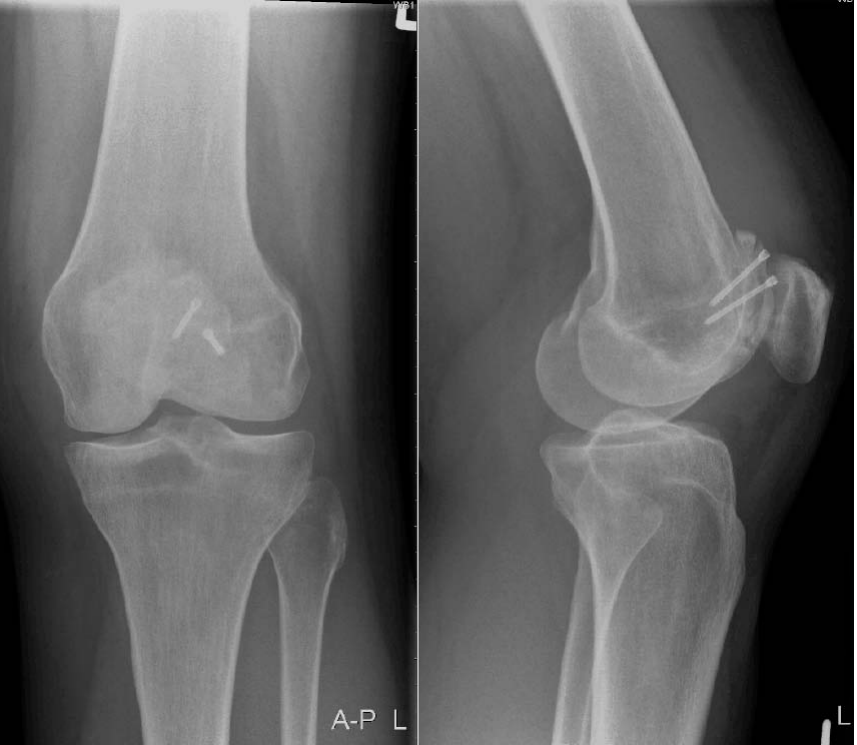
Radiograph of the left knee 5 months after trochleaplasty.
